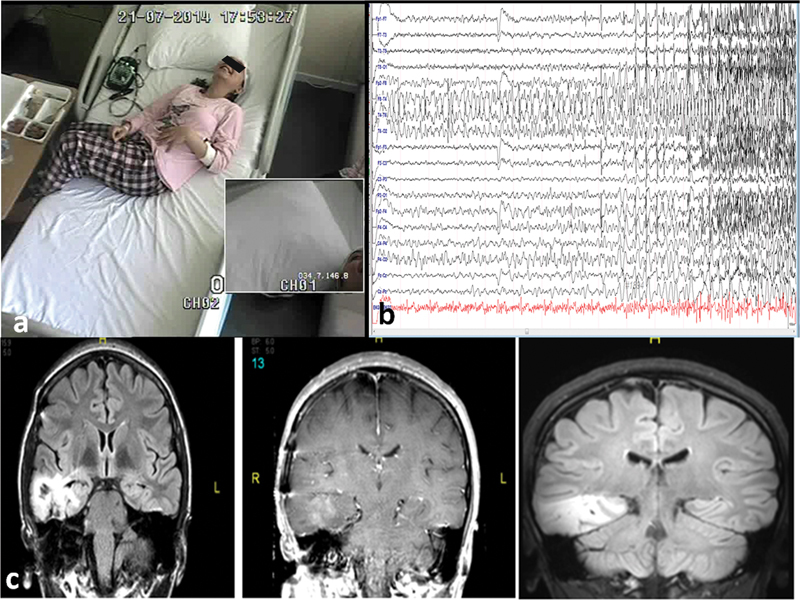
Patient 31: a) Crying-laughter without mirth, b) ictal EEG: F8-T4 sharp waves, c) cranial MRI, right temporal residual tumour.
